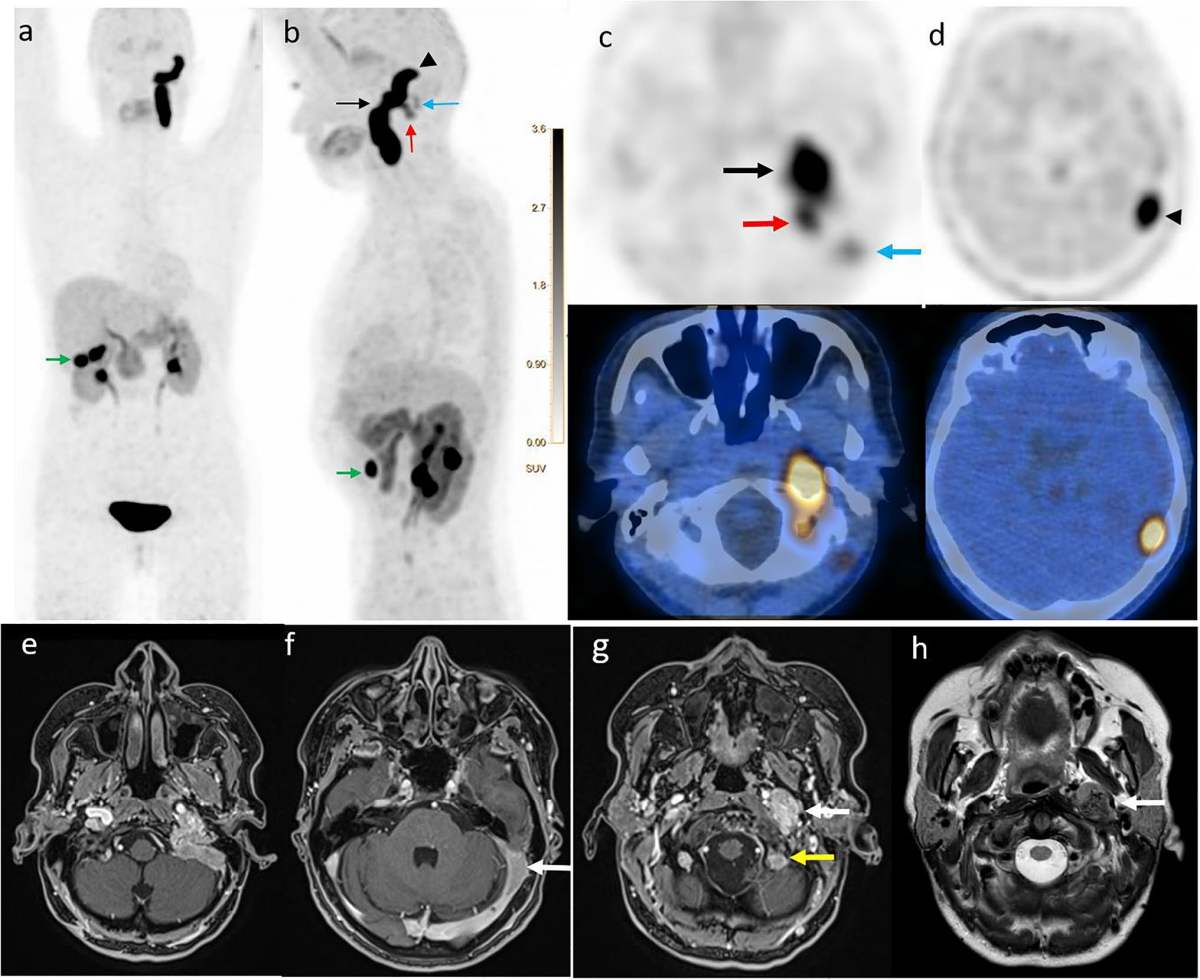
a. [18F]FDOPA PET: MIP (Maximum Intensity Projection) anterior view shows intensive tracer uptake along the left cervical and intracranial regions. b. [18F]FDOPA PET/: MIP left lateral c–d. [18F]FDOPA PET (upper row) and fused PET/CT axial images (lower row) show intensive tracer uptake in the area of the left jugular foramen (b–c, black arrow) with cranial extension to the ipsilateral sigmoid sinus, and condylar emissary vein (b–c, red arrow), and an intracranial extension along the left cerebellar hemisphere to the lateral aspect of cerebellar tentorium (b–d, arrowhead). In the caudal dimension, the tumoral tissue shows intensive tracer uptake along the cervical vascular nerve sheath to the level of the hyoid bone. In addition, a small tumoral extension with faint uptake is also seen in the splenius capitis muscle region (b–c, blue arrow). e–h. Magnetic Resonance Imaging (MRI) axial T1 fat suppression with intravenous Gadolinium (e–g), axial T2 TSE (h) demonstrating large tumour expansion centred at the jugular foramen with local bony destruction. Contrast enhancement with typical salt and pepper-appearance with multiple internal flow voids are best seen in T2. Tumoral extension is evident in the sigmoid sinus (f, arrow), the condylar emissary vein via the condylar canal (g, yellow arrow) as well extra cranially along the internal jugular vein with encasement of the internal carotid artery (g–h, white arrow)
